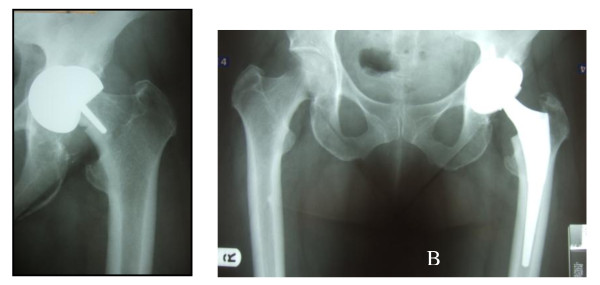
A- Preoperative X-ray study showing gross loosening of the socket with a femoral neck fracture; B- Post operative X-ray six months later.
